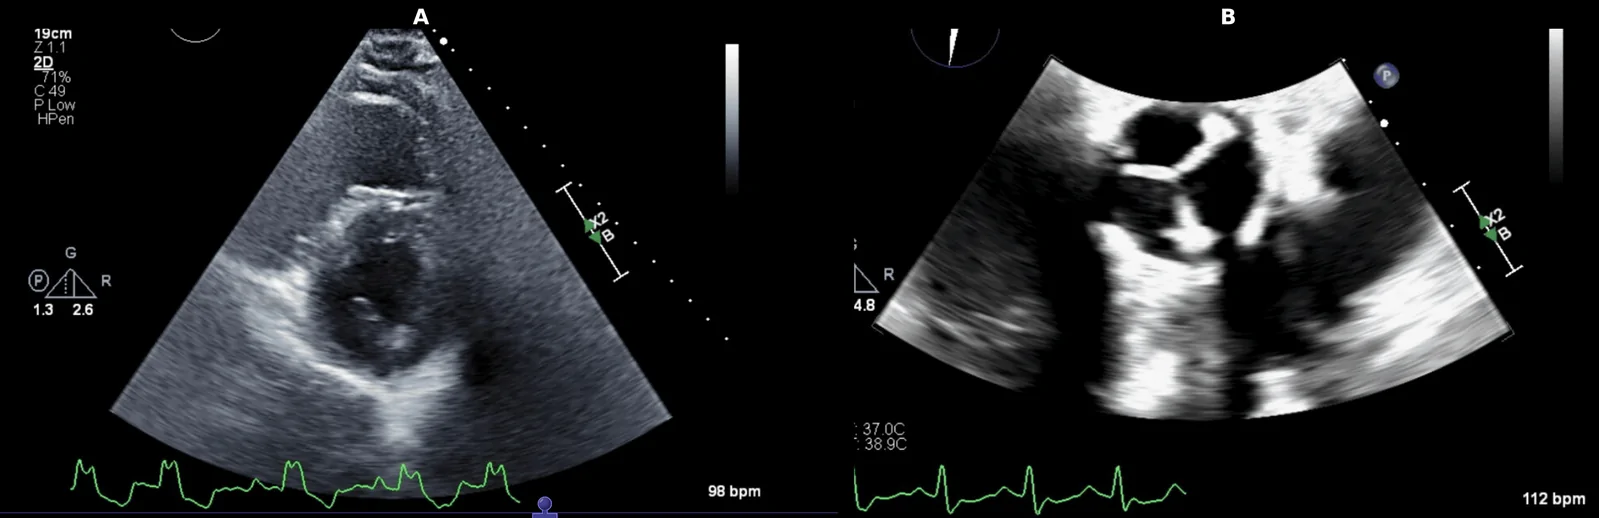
Imaging findings (second admission) Repeat transthoracic (A) and transesophageal (B) echocardiography, again without echocardiographic evidence of vegetation or periannular involvement.
